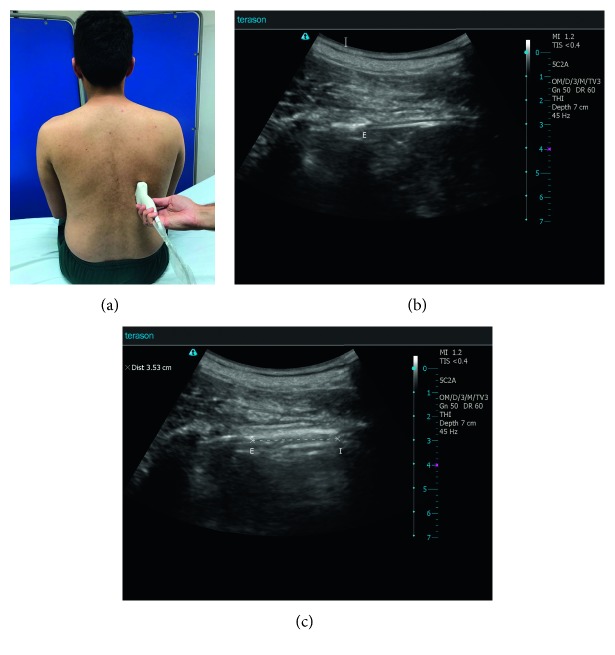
(a) Sonographic measurement of the upward and downward movements of the lung silhouette, here on the right side (lung silhouette method). The patient is sitting; the transducer is placed at the lowest point of the lung silhouette in the scapular line. While the patient breathes as deeply as possible, a video sequence is performed. Afterward, the distance between maximal inspiration and maximal expiration can be measured. (b) Sonographic measurement of the upward and downward movements of the lung silhouette, here on the right side. E marks the lowest point of the lung silhouette at maximal end expiration. (c) Sonographic measurement of the upward and downward movements of the lung silhouette—here on the right side. E marks the lowest point of the lung silhouette at maximal end expiration, and I marks the lowest point at maximal inspiration. In this example, the distance between E and I is 35.3 mm.
